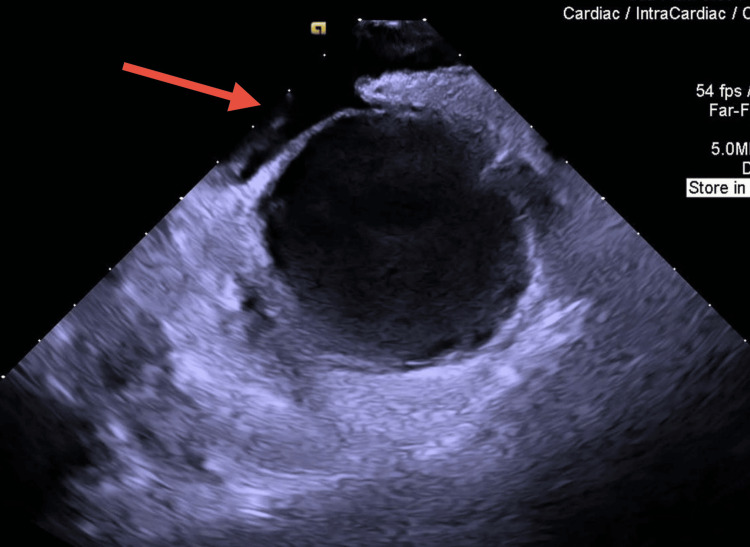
Intracardiac echocardiography (ICE) image showing a patent foramen ovale The septum primum flap is seen separating from the septum secundum, creating a pressure-dependent right to left shunt pathway.
